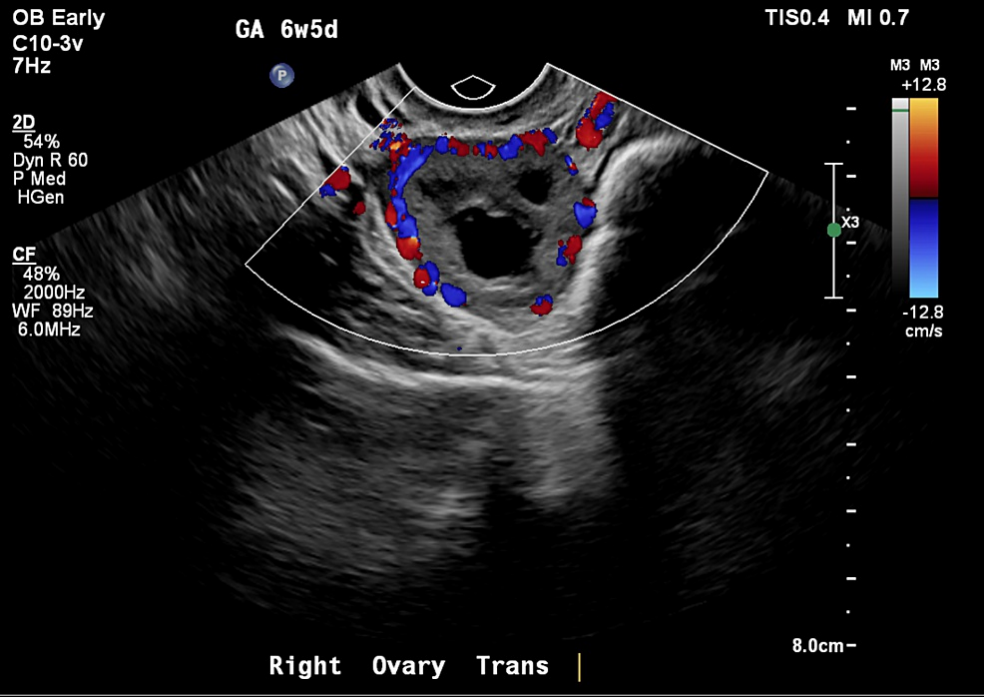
"Ring of Fire" on ultrasound
